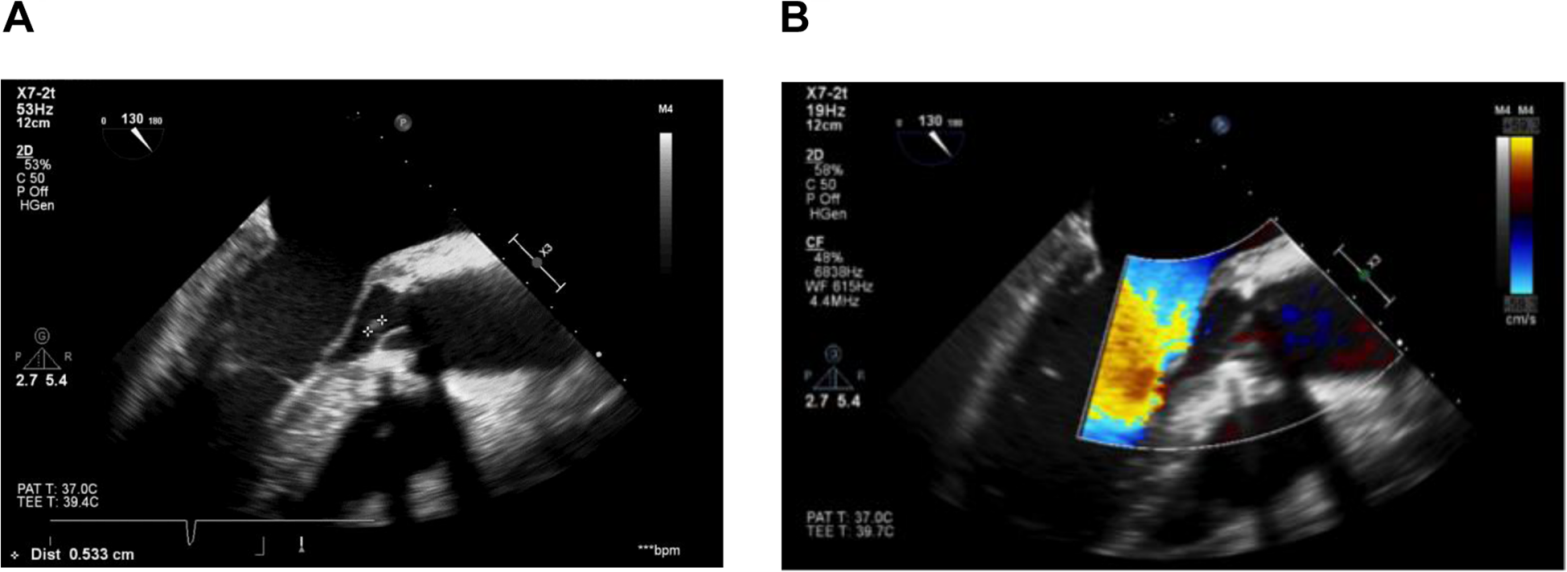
Trans-oesophageal echocardiography (TOE) showing (a) location and size of vegetation and (b) mitral regurgitation
